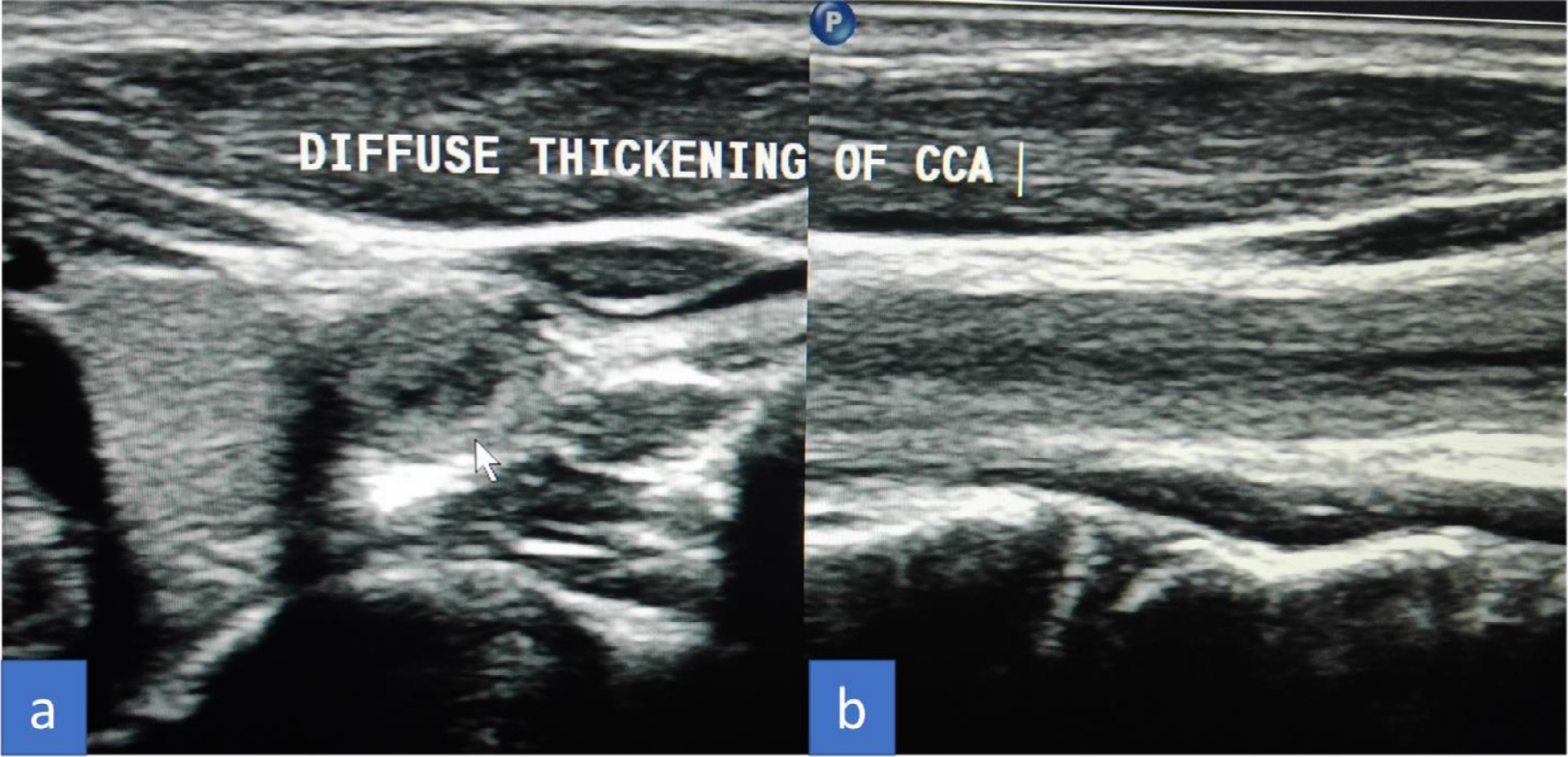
Ultrasonography of common carotid artery (CCA) transverse image (A), longitudinal image (B) showing diffuse circumferential thickening of CCA.
